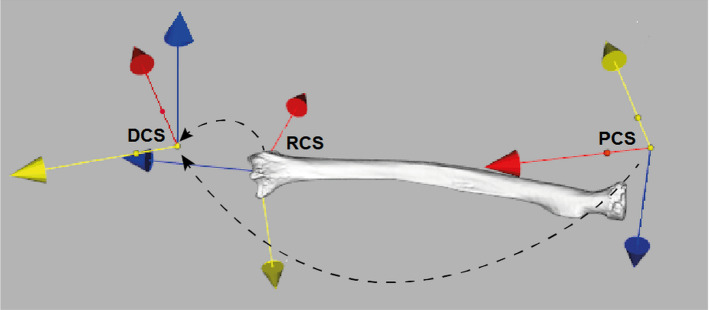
For each CT image, after segmentation, three Coordinate Systems (CS’s) are defined: an anatomical CS for the radius bone (RCS) and CS’s for the distal and for the proximal marker tools (DCS and PCS) on the cast. The transformation from RCS-to-DCS provides the relative position of the radius with respect to the cast. The transformation from PCS-to-DCS represents the cast deformation
